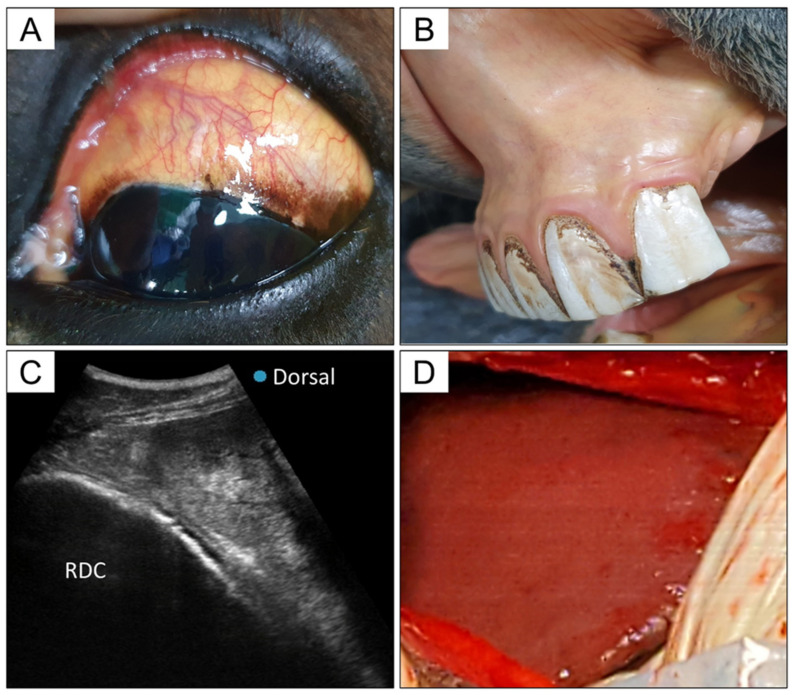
Clinical findings of Theiler’s disease case seen in this study. (A) Yellowish color change in the sclera. (B) Yellowish color change of mucous membrane. (C) Ultrasonographic image of the liver obtained from the right abdomen. The liver shows heterogeneous and irregular surface with a hyperechogenic structure in the liver parenchyma; RDC, right dorsal colon. (D) Liver appearance during laparotomy. The liver has remarkably blunt edges and irregular surfaces with dark-reddish color changes.
